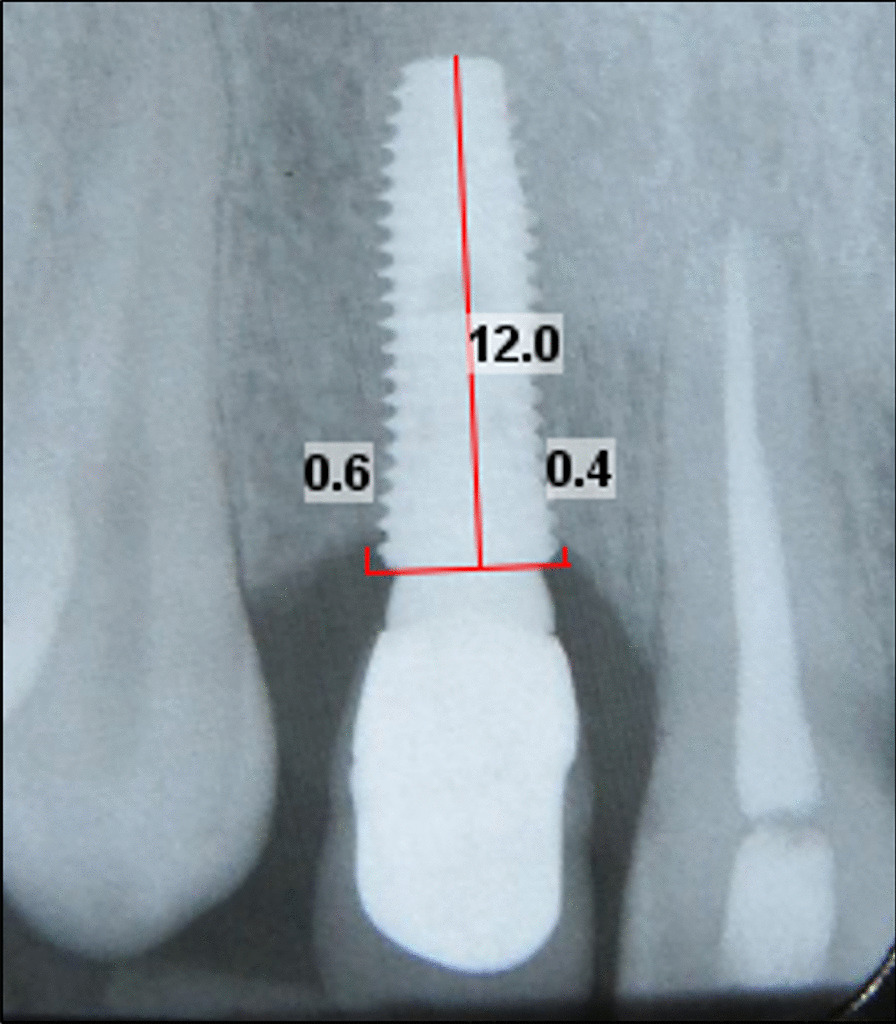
After calibration of radiographs by the length of each implant, marginal bone loss measured as vertical distance between implant shoulder and the marginal bone
